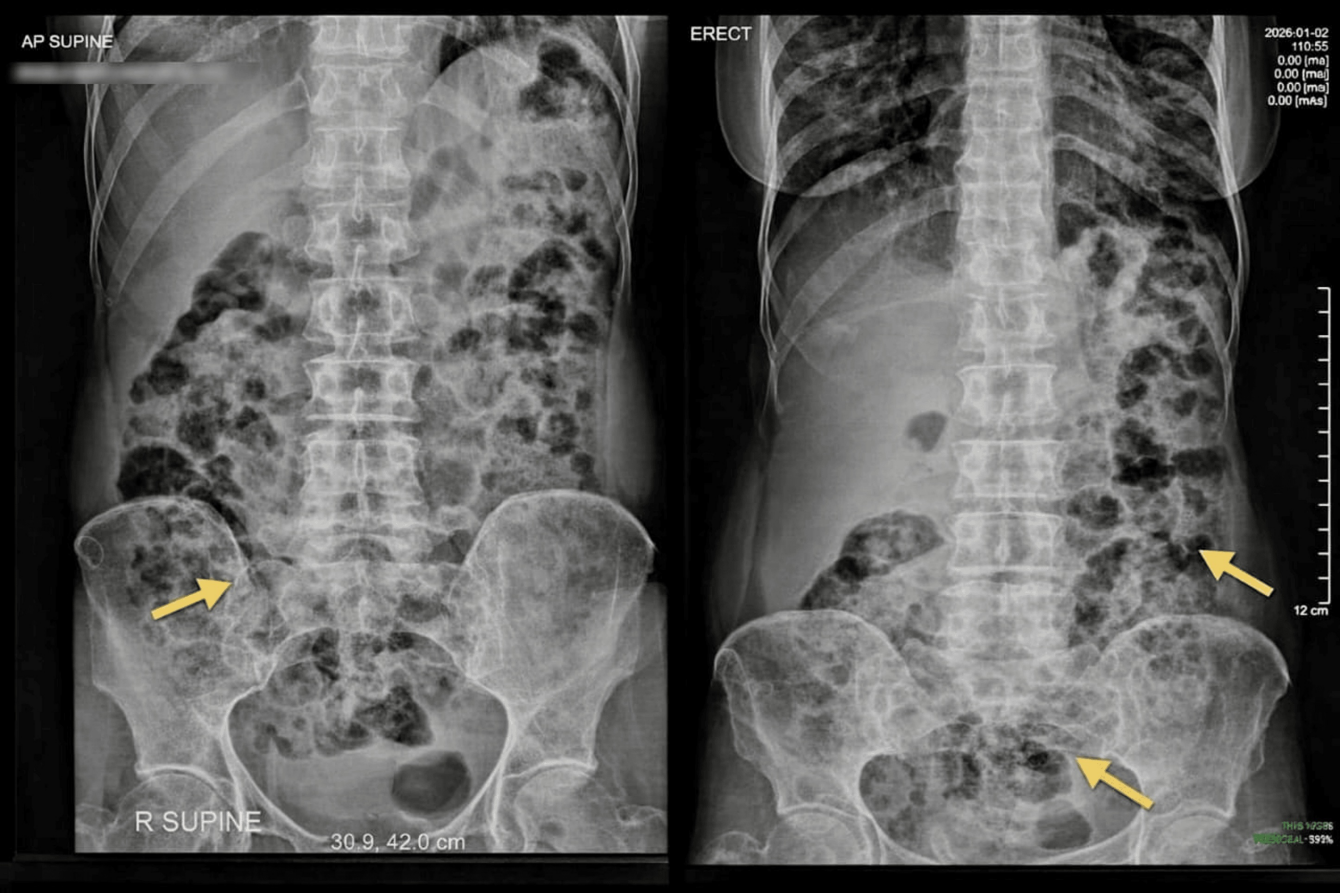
Abdominal radiographs demonstrating colonic fecal loading. Supine and erect abdominal radiographs show diffuse colonic gaseous distension with prominent fecal loading (arrows). There are no air-fluid levels, free sub-diaphragmatic air, or radiographic features of a transition point.
